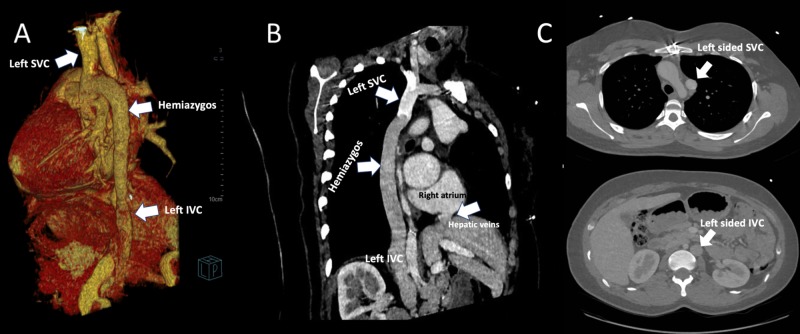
Volume rendered and maximum intensity CTA A) Volume rendered chest CTA showing the left IVC with hemiazygos continuation draining to the left-sided SVC. (B) Oblique coronal maximum intensity CTA image of the chest demonstrating the left hemiazygous arch. The hepatic veins drain directly into the right atrium. The branch from the left SVC draining into the coronary sinus is not included in the image. (C) Axial CTA images showing left-sided SVC and left-sided IVC. CTA, computed tomography angiography; SVC, superior vena cava; IVC, inferior vena cava
